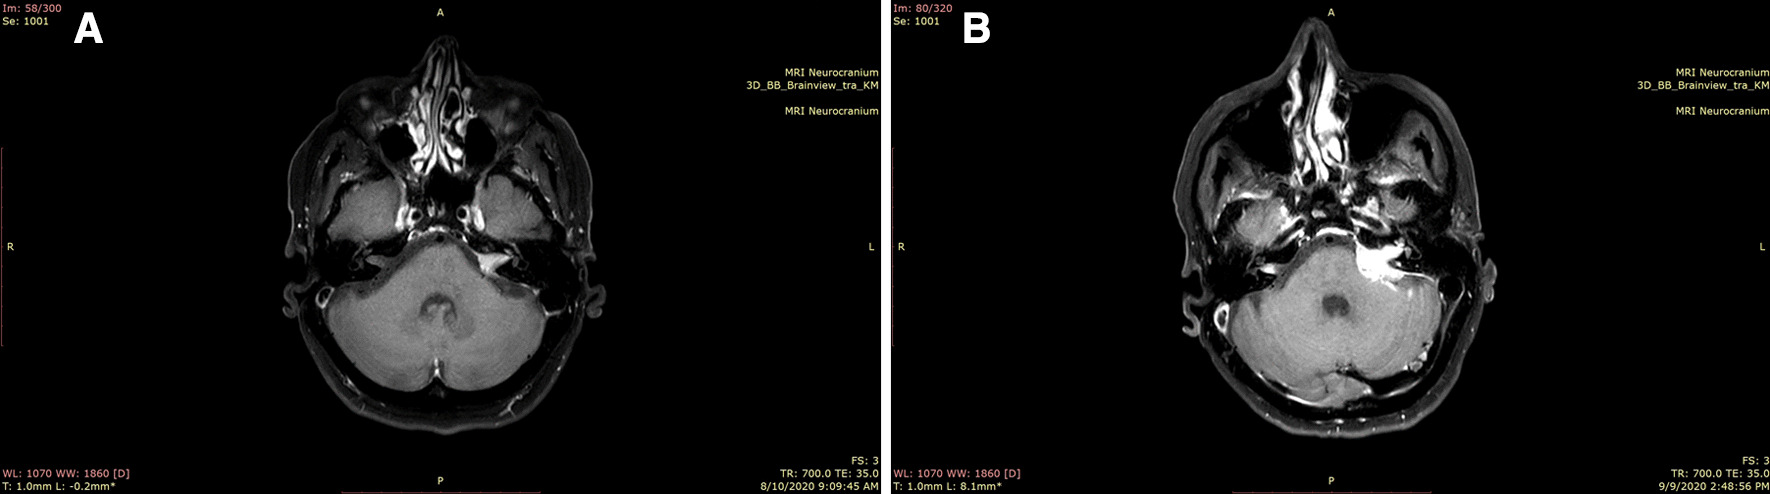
Brain magnetic resonance imaging of lesions in the left internal acoustic channel advancing with schwannoma-like growth 2 and 4 months after diagnosis. a T1-weighted cranial magnetic resonance imaging after contrast showing the tumor in the left internal acoustic channel at 2 months post-surgical follow-up. b T1-weighted cranial magnetic resonance imaging after contrast revealing infiltrative tumor growth with suspicious leptomeningeal involvement in the cerebellopontine angle within 4 months after diagnosis. Right nasal fracture upon fall is shown
